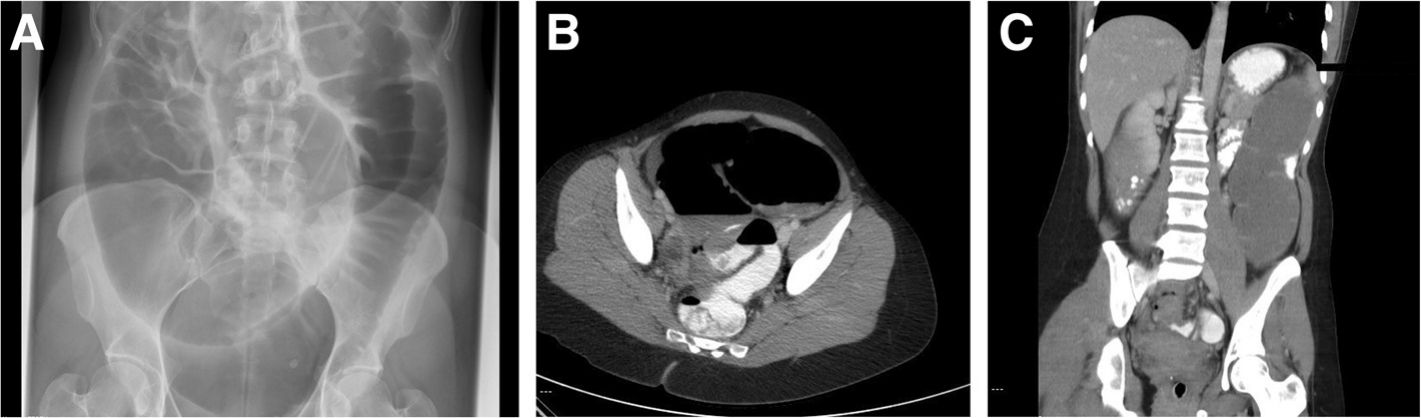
Pre-operative imaging studies. X-ray (A) and computed tomographic scans (B, C) of the patient’s abdomen show a significantly inflated cecum and an obstruction in the sigmoid colon with a proximal distension of the colon.
